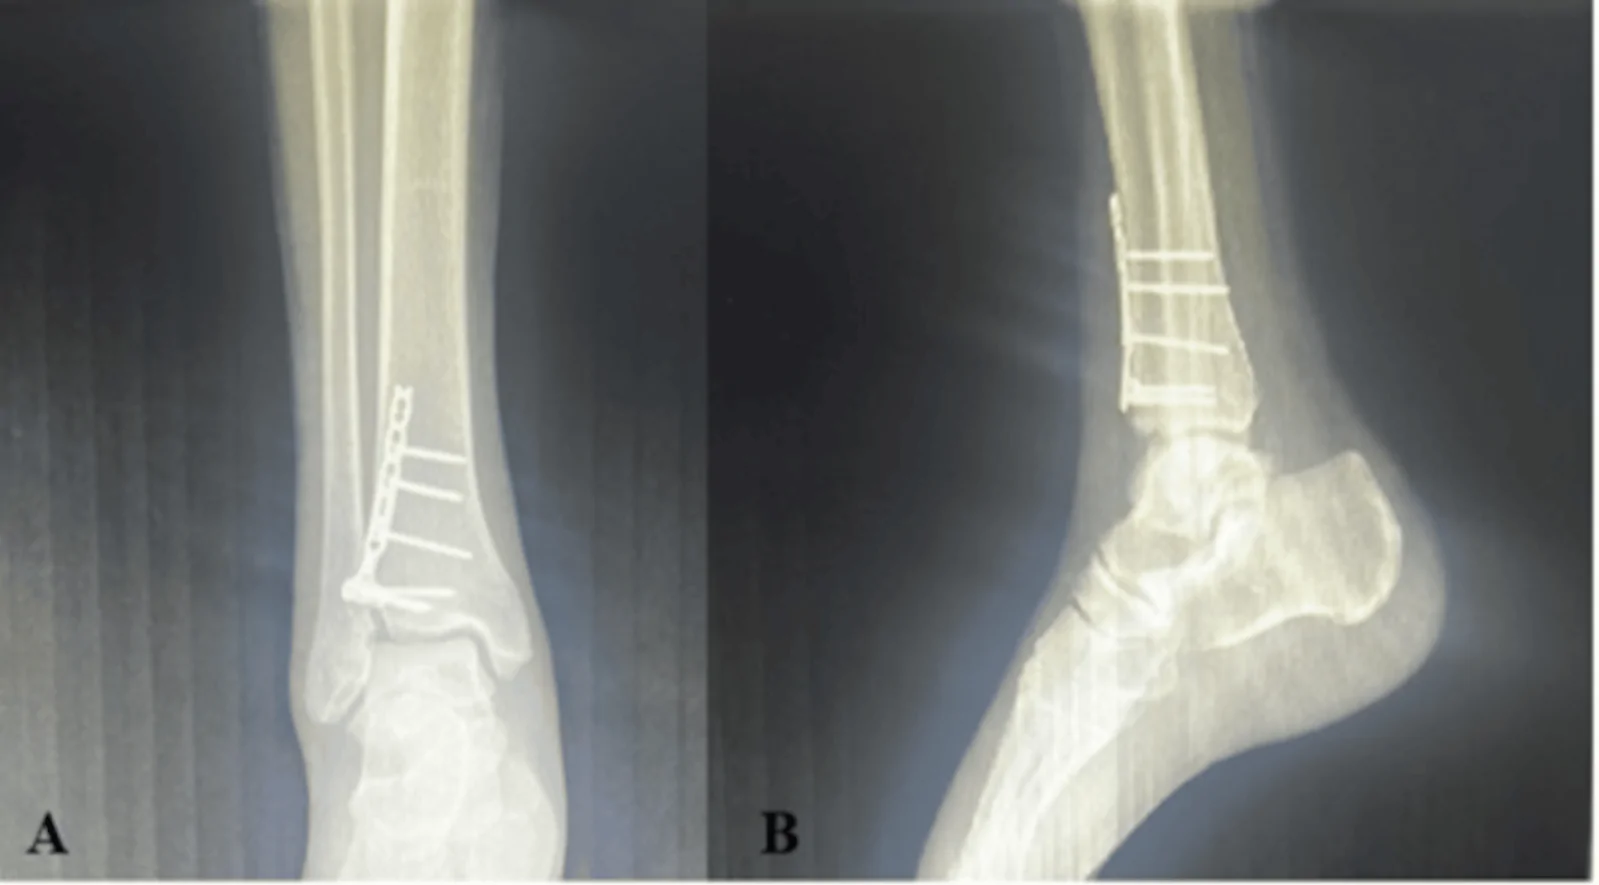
Follow-up X-rays. Three-month follow-up ankle anteroposterior (A) and lateral (B) X-ray views showing complete healing of the fracture without further displacement or disruption of the joint congruency.
